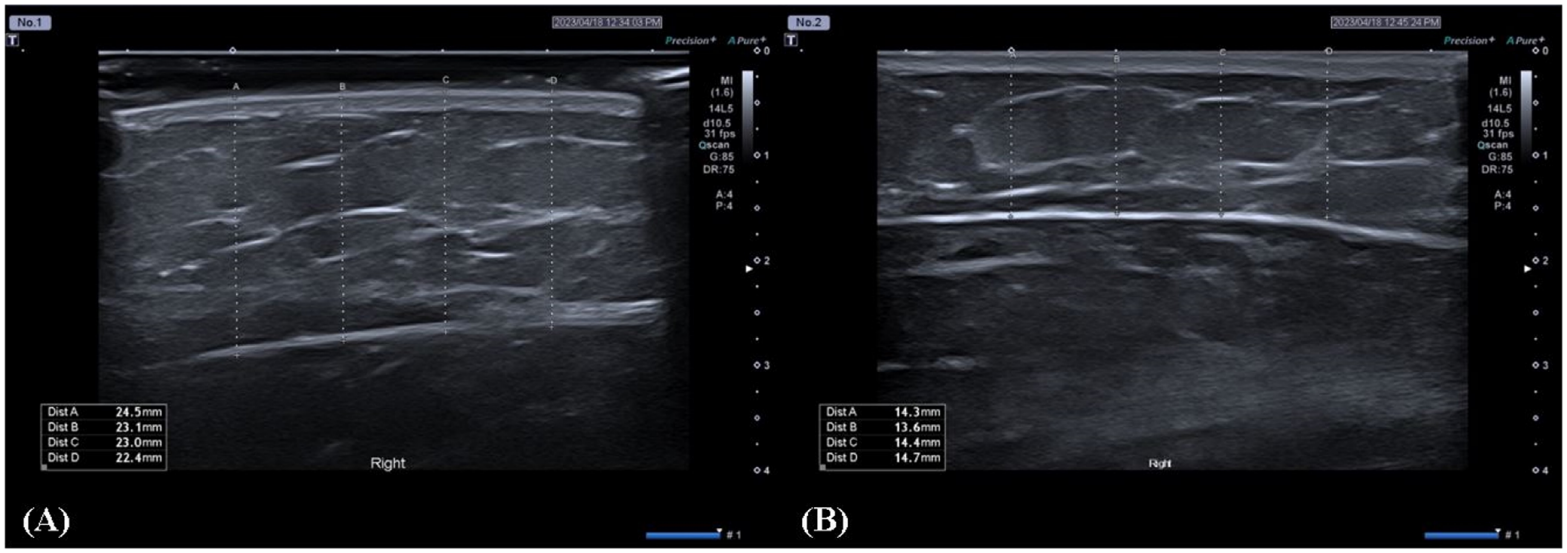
Ultrasound imaging of the arm (A) before and (B) 20 weeks after treatment with a 5 mg/mL V‐OLET injection.
